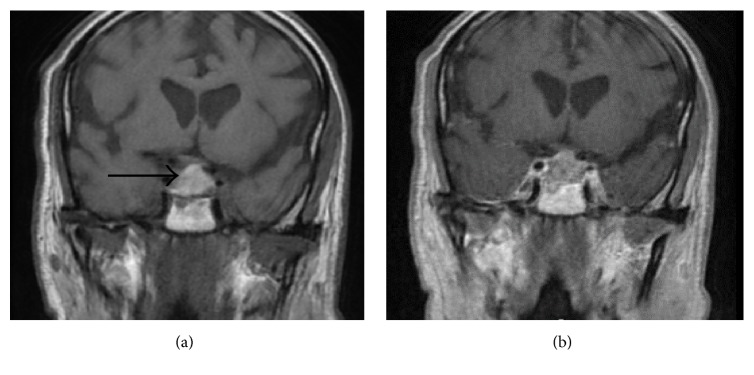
Brain MRI W/WO contrast 2 days before surgery. (a) Coronal T1-weighted image demonstrates a large sellar mass with suprasellar extension and slight mass effect on the optic chiasm. This mass exhibits marked hyperintense signal on T1-weighted image (arrow), which indicates blood products and recent hemorrhage. (b) Coronal T1-weighted postcontrast image shows normal enhancement of the cavernous sinus. No enhancing mass or nodule is evident in association with intrinsically T1 bright lesion. The mass abuts approximately 90 degrees of the right internal carotid artery contour. This abutment is less on the left side. The carotid arteries are patent and show normal caliber without any narrowing. No normal pituitary tissue is identified.
